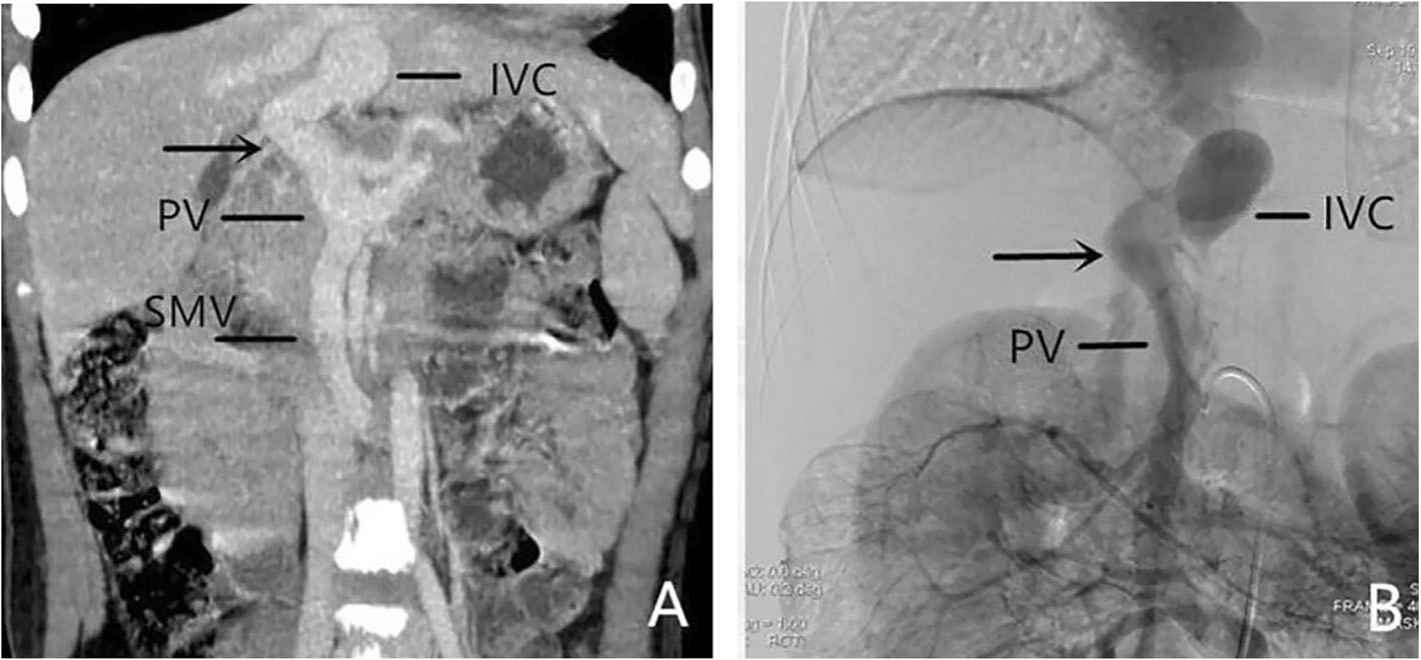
Female, 11 years old, Abernethy Ib. a: Abdominal CT angiography maximum intensity projection (MIP) image (portal phase) shows the presence of extrahepatic portosystemic shunt (arrow) with absence of intrahepatic portal veins. b: DSA image confirmed the MSCT result
